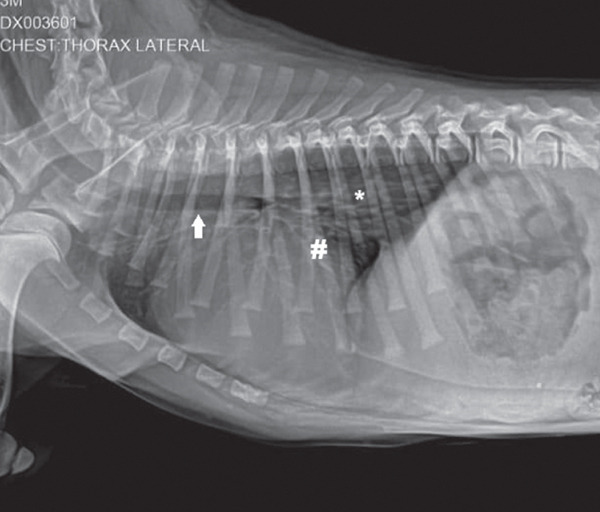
LLRL chest radiograph. Dorsal displacement of the trachea (∗), globular cardiac silhouette (#), interstitial and bronchial pattern (arrow) in caudal pulmonary lobes, suggestive of generalized cardiomegaly and bronchopneumonia.
